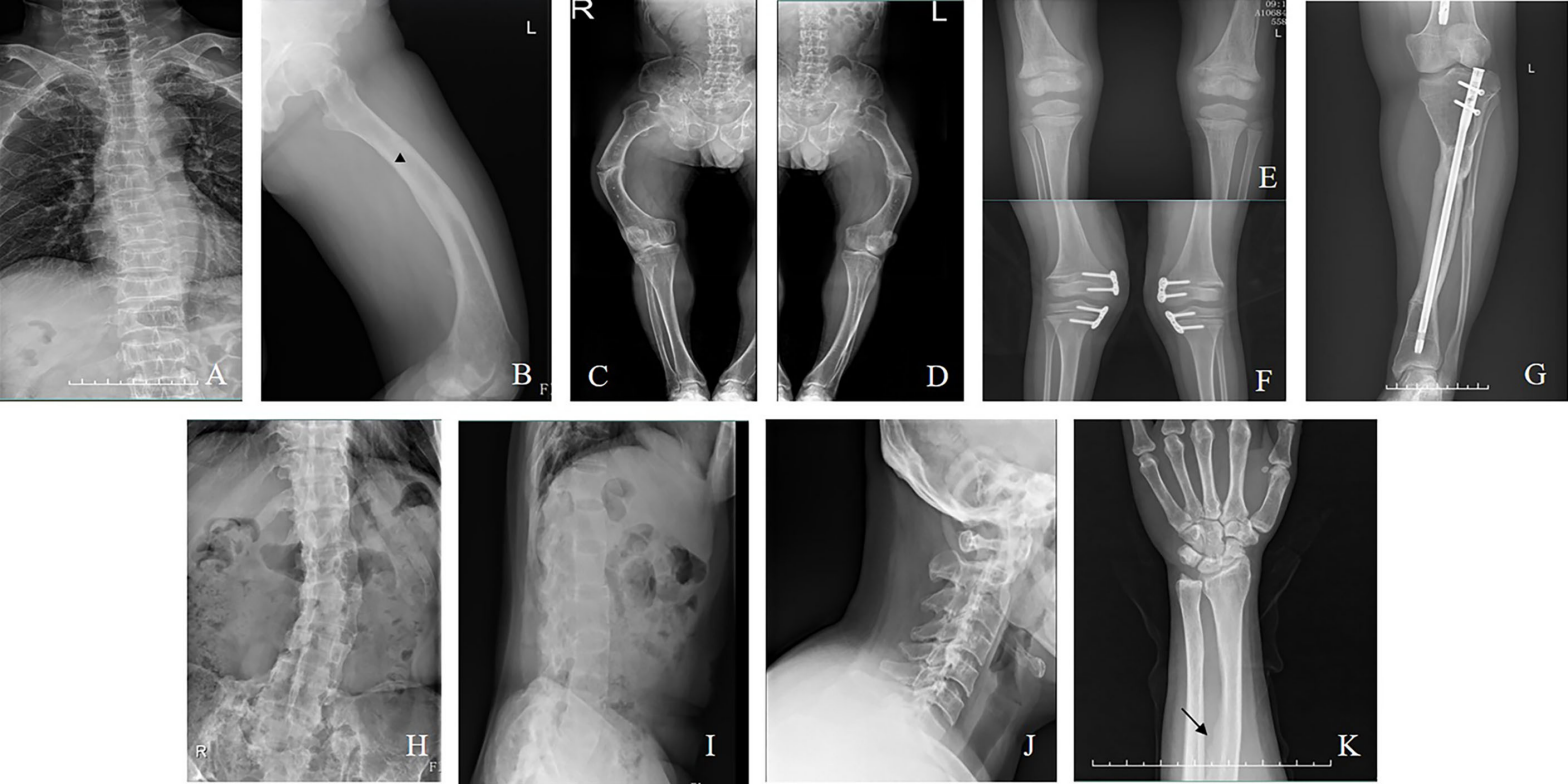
(A) Vertebral compression fractures (Family 5/III-8, a man aged 42). (B): Pseudofacture line (Family 4/III-17, a man aged 22). (C, D) Fractures in the femur (Family 5/III-8). (E) Radiograph of bilateral knee (Family 2/II-4, a boy aged 5). (F) Radiograph after hemiepiphysiodesis (Family 9/II-3, a girl aged 7). (G) Radiograph after osteotomy (Family 14/III-6, a woman aged 24). (H–J) Ossification of the spinal ligaments (Family 14/II-3, a woman aged 50). (K) Ossification of the interosseous membrane of the forearm (Family 6/II-4, a woman aged 30).
